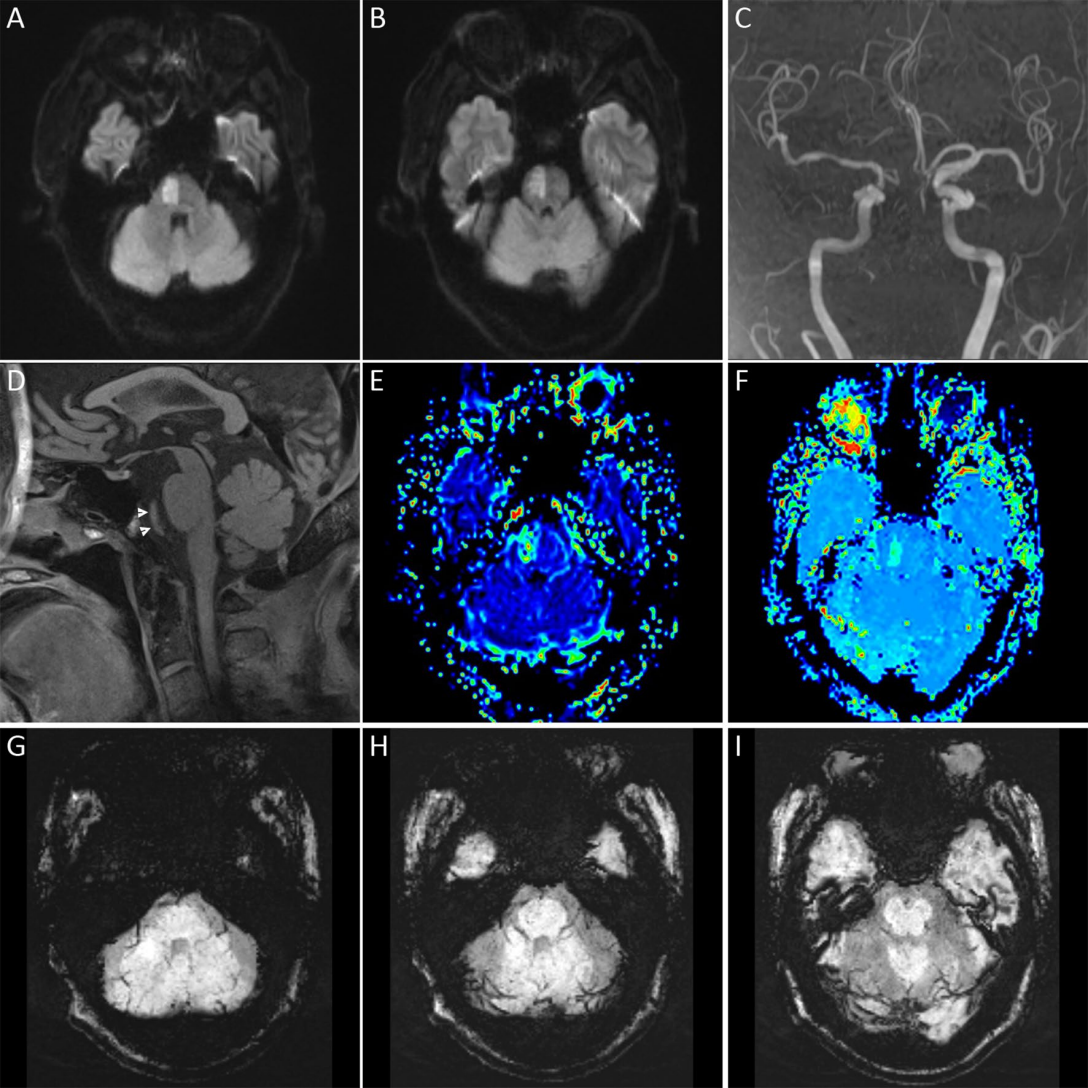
Prominent vessel sign was not displayed in a patient with posterior circulation occlusion. DWI (A,B) showed acute pontine infarction. MRA (C) and 3D-SPACE (D) indicated occlusion of the basilar artery (white arrowheads). The MTT (E) and TTP (F) maps showed hypoperfusion at the infarct site. There was no asymmetric prominent hypointense vessels on SWI (G–I) in the posterior circulation territory.
